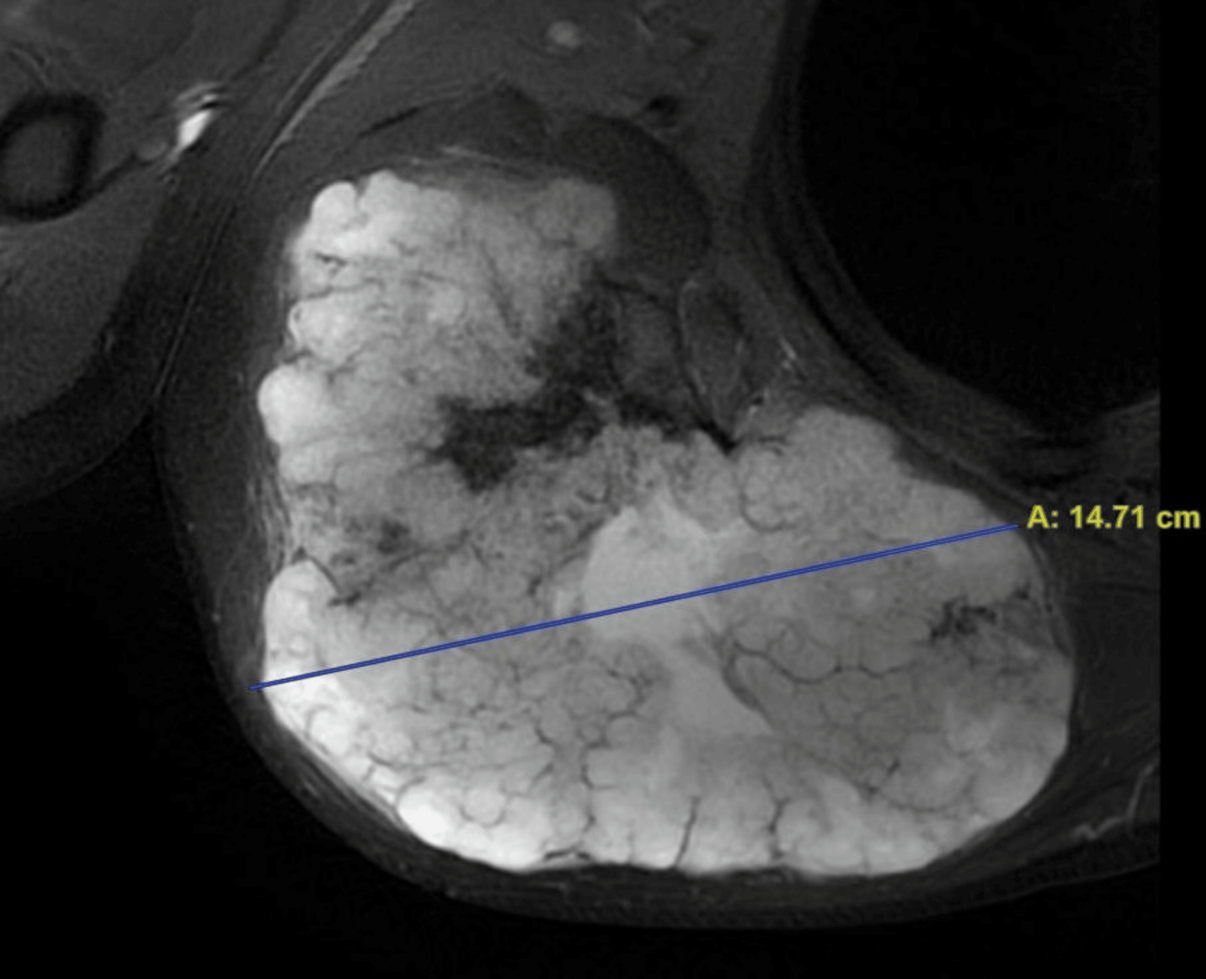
Preoperative axial MRI view. The tumor measured 14.71 cm in the maximum medial-to-lateral axis.
